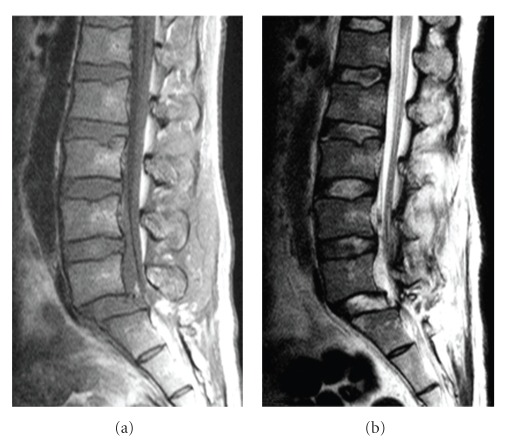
Sagittal magnetic resonance imaging showing anterior displacement of L5 on S1 and disruption of the posterior ligamentous complex. (a) T1-weighted images. (b) T2-weighted images.
